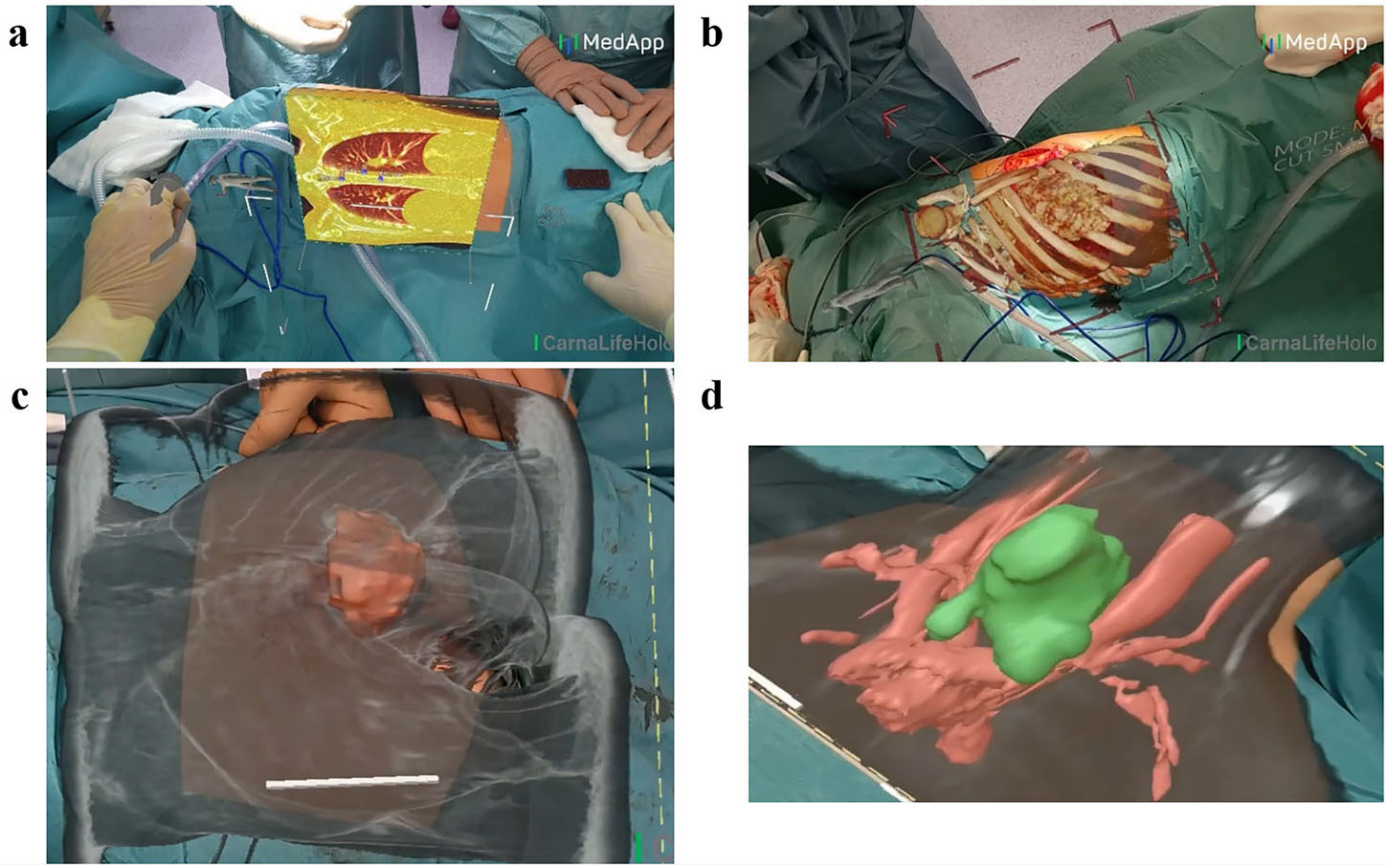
Intraoperative visualization using a hologram overlay. The holographic model, generated from preoperative Computed Tomography (CT) and Magnetic Resonance Imaging (MRI) data, is aligned with the patient's anatomy by detecting radiological markers and using voice commands and hand gestures. A printed reference point ensures stable positioning throughout the procedure, allowing realignment if the overlay is lost. The system enables real-time visualization of internal anatomical structures, assessment of critical elements such as major blood vessels, and intraoperative measurements without breaking the sterile field. The figure presents surgical procedures using MR-assisted intraoperative visualization: (a) thoracotomy, (b) resection of a chest tumor, (c) resection of a tumor of the left clavicle, and (d) resection of a sternal end clavicular tumor.
